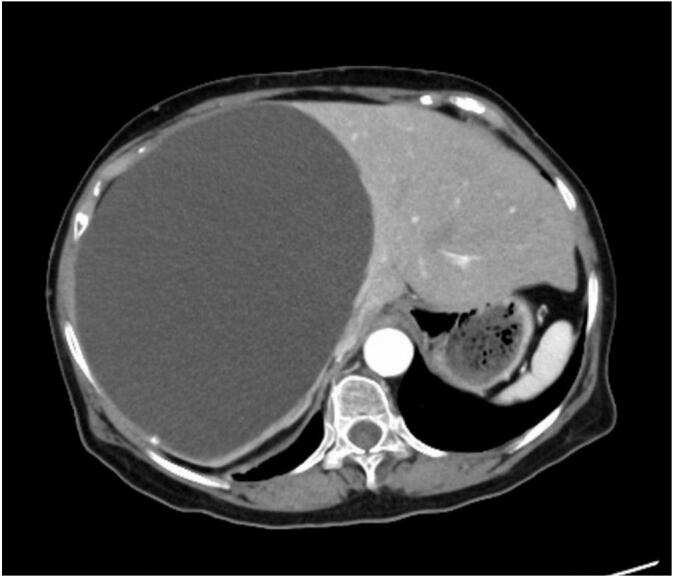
A large hepatic cyst. An 82-year-old woman came to our hospital because of abdominal distention. An abdominal computed tomography (CT) scan showed a large hepatic cyst.
